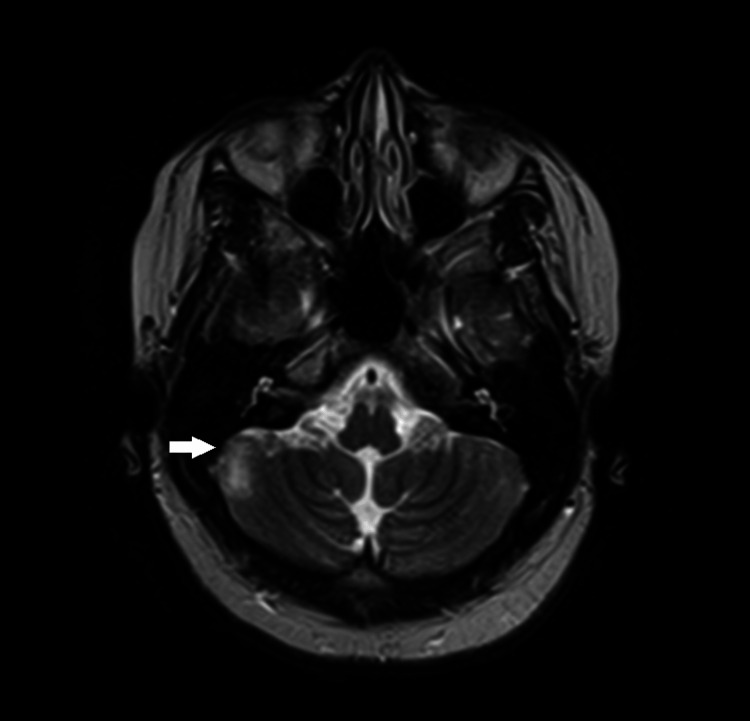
Axial MRI showing FLAIR signal hyperintensity (white arrow) in the right aspect of the cerebellum. MRI: magnetic resonance imaging; FLAIR: fluid-attenuated inversion recovery
